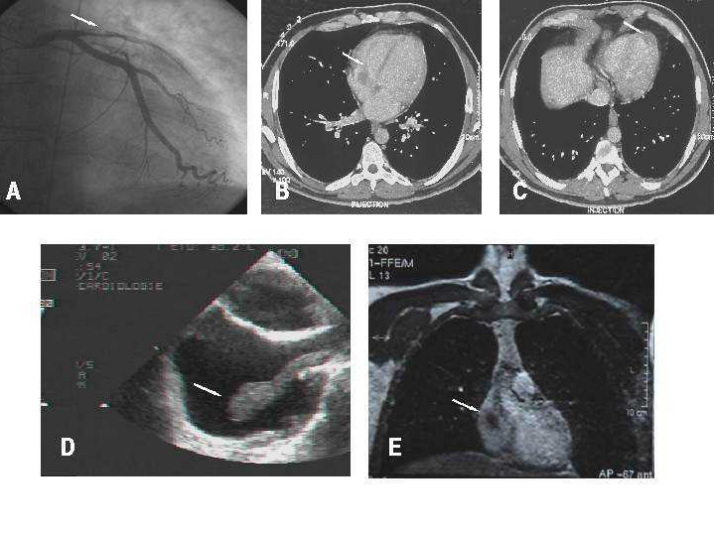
At patient admission, left coronary angiogram revealed thrombotic obstruction of left anterior descending artery (A). Large thrombus in the right auricle emerging through tricuspid valve within right ventricle (B) and apical thrombus complicating anterior aneurysm (C) detected by computer tomography scan, performed the next day. Large thrombus emerging from superiour vena cava and prolabing in the right auricle, evidenced by Trans-oesophagial echocardiography (D). Extensive thrombus emerging from superiour vena cava within right auricle, evidenced by magnetic resonance imaging (E).
